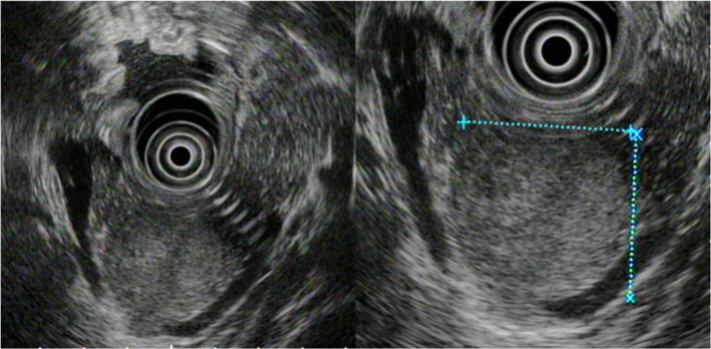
Endoscopic ultrasound. These two images show a low echoic mass of 35mm diameter in the pancreatic tail. (The left image shows a whole image and the right image shows an enlarged image. The dotted lines show lines of measurement for this tumor.)
